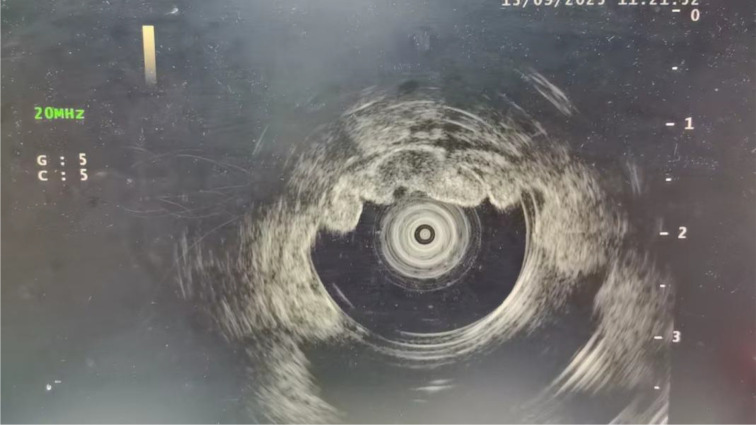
Endoscopic ultrasound showed thickening of the mucosal layer, with clear demarcation between the tumor and the muscularis propria.
